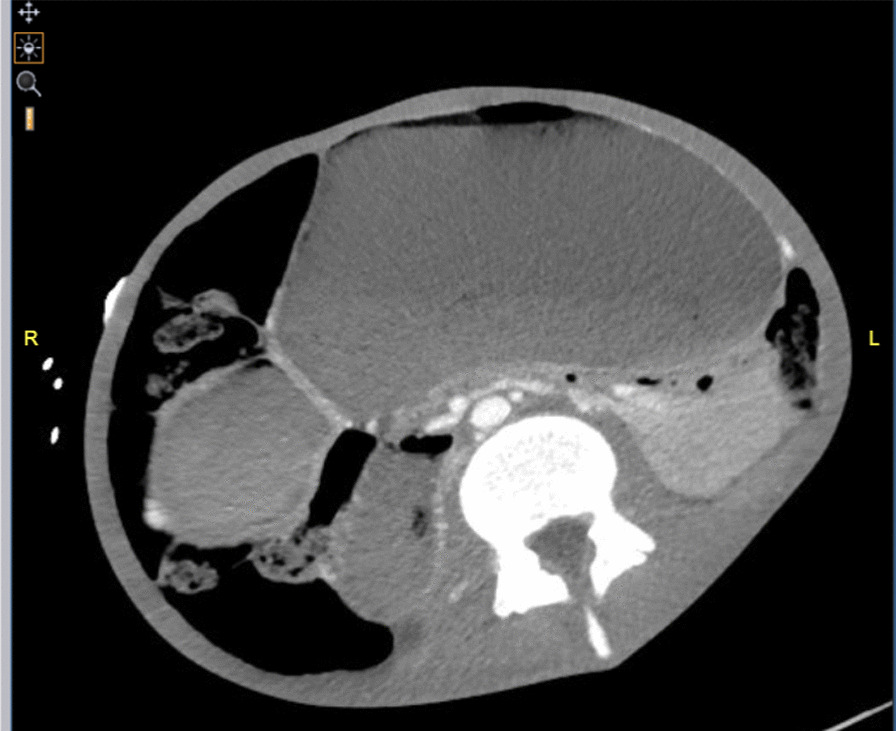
Computed tomography transverse view
